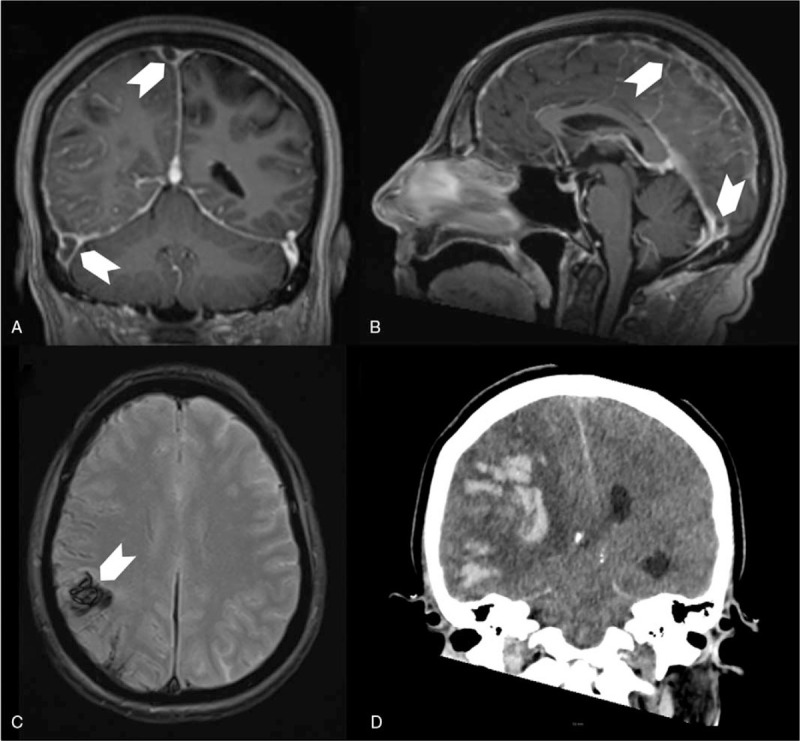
Patient (case 1) cerebral imaging showing cerebral venous thrombosis and venous infarction with hemorrhagic transformation. A (frontal view), and B (sagittal view): cerebral MRI T1 sequence with gadolinium showing filling defect in the superior sagittal sinus and the right lateral sinus (arrows), C (axial view): T2∗-weighted gradient-recalled echo resonance imaging showing right parietal hemorrhage (arrow), D (frontal view): 24-hour control cerebral CT scan showing extended venous infarction with hemorrhagic transformation leading to subfalcine and transtentorial herniation.
